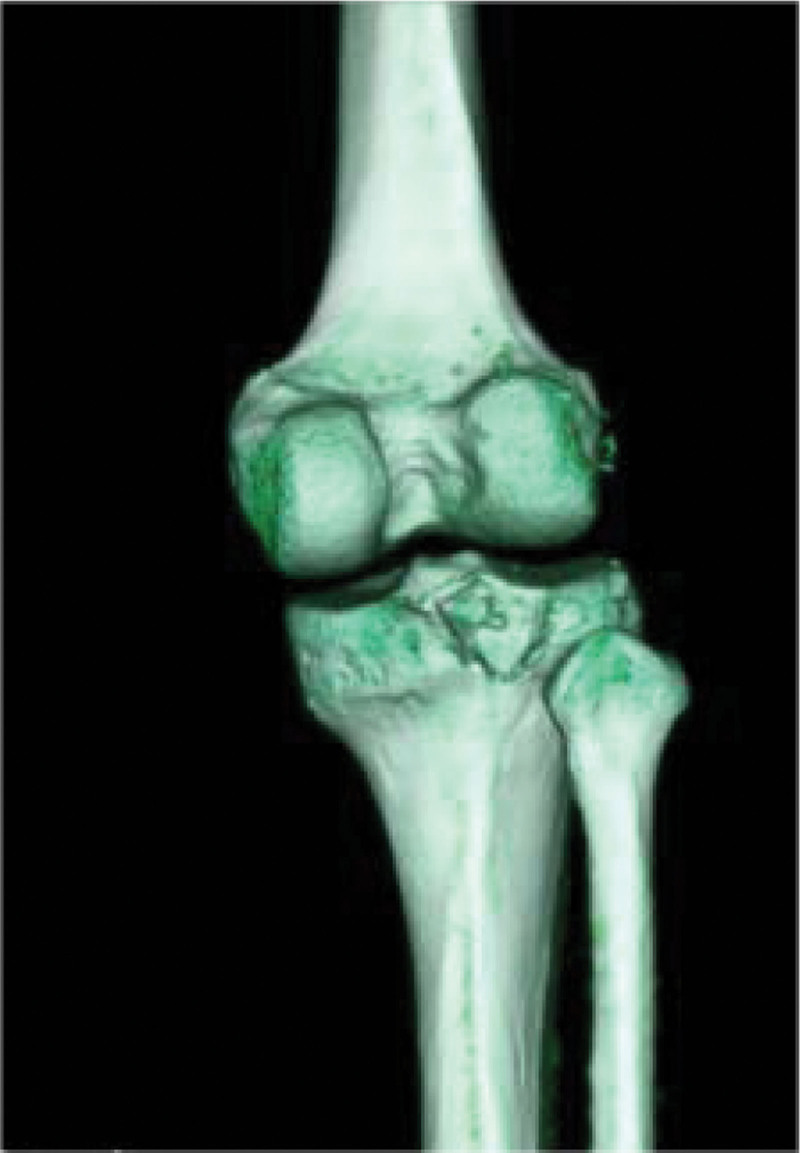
Postoperative three-dimensional CT image. CT = computed tomography.
